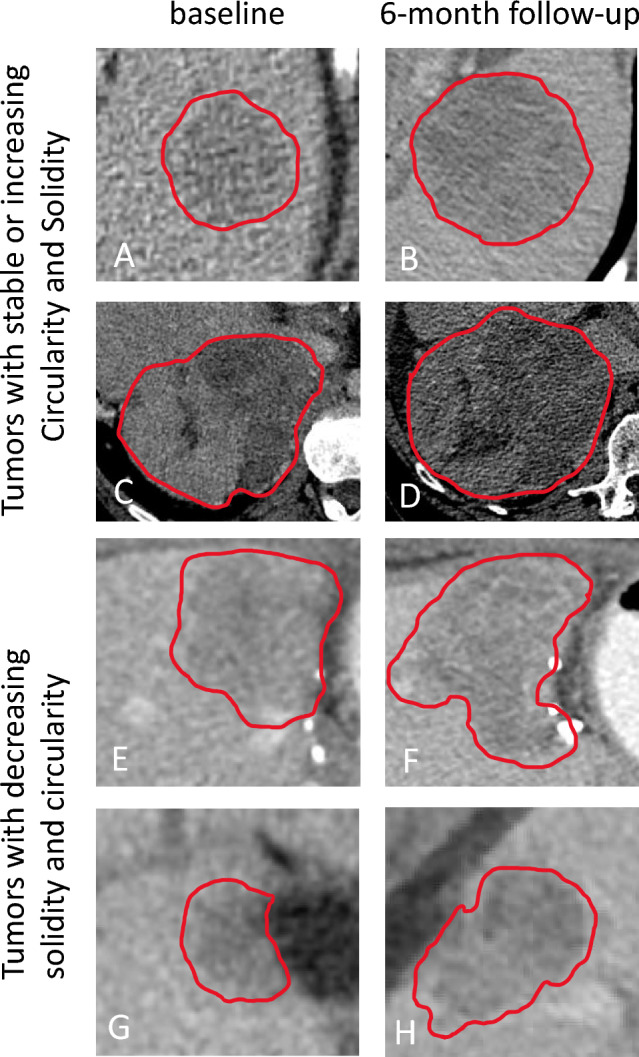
Four representatives cases showing the evolution of tumor shape between baseline (left) and 6-month follow-up (right). Tumors were manually segmented on axial CT images (red line). The two upper cases, (A–D) are tumors with stable or increasing solidity and circularity. The two lower cases, (E–H) are tumors with decreasing solidity and circularity (becoming more irregular and less circular tumors). Hepatocellular carcinoma (A, B) solidity: 0.96 then 0.97, and circularity 0.88 then 0.86. Adrenal metastasis of a hepatocellular carcinoma (C, D) solidity: 0.98 then 0.99 and circularity: 0.86 then 0.93. Cholangiocarcinoma (E, F) solidity: 0.95 then 0.82 and circularity: 0.76 then 0.58. Cholangiocarcinoma (G, H) solidity: 0.93 then 0.91 and circularity 0.80 then 0.64.
